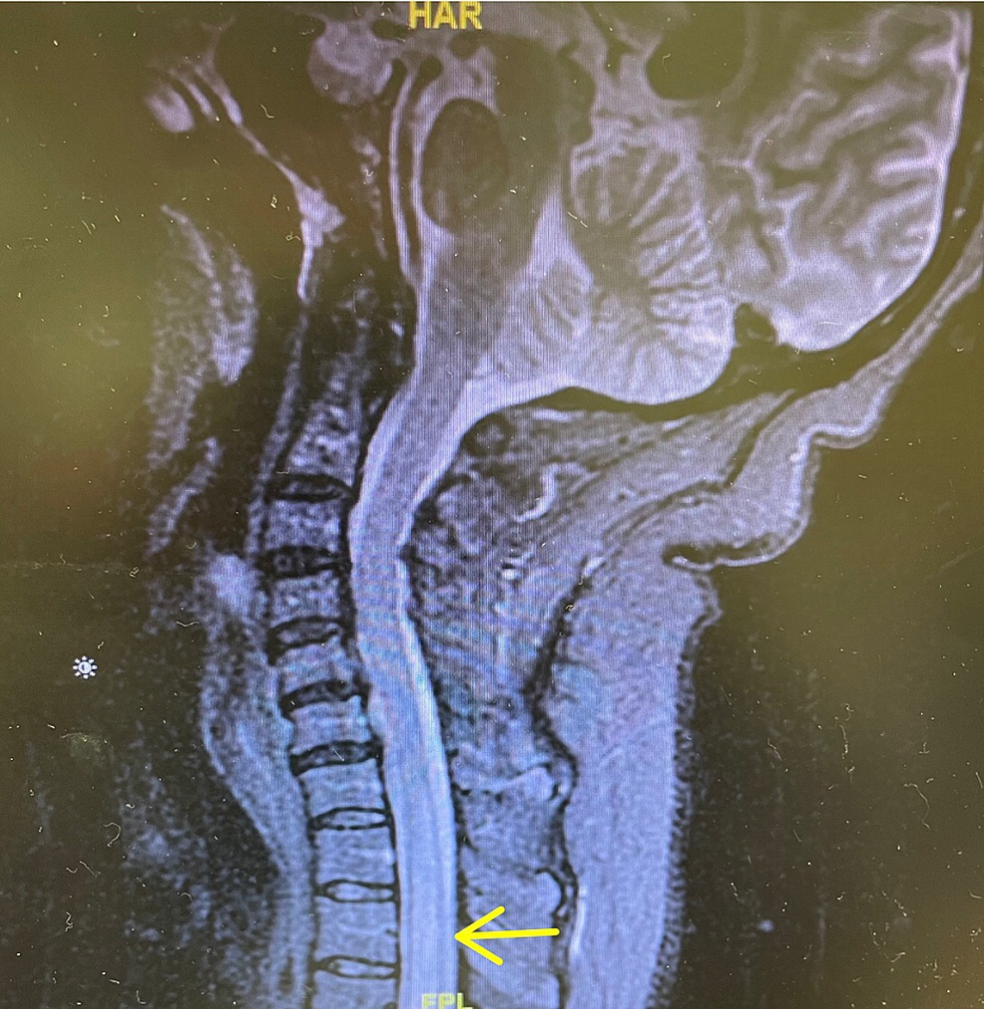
T2-weighted cervical spine MRI showing hyper-intense intramedullary lesion beginning at the level of C7 (yellow arrow).
